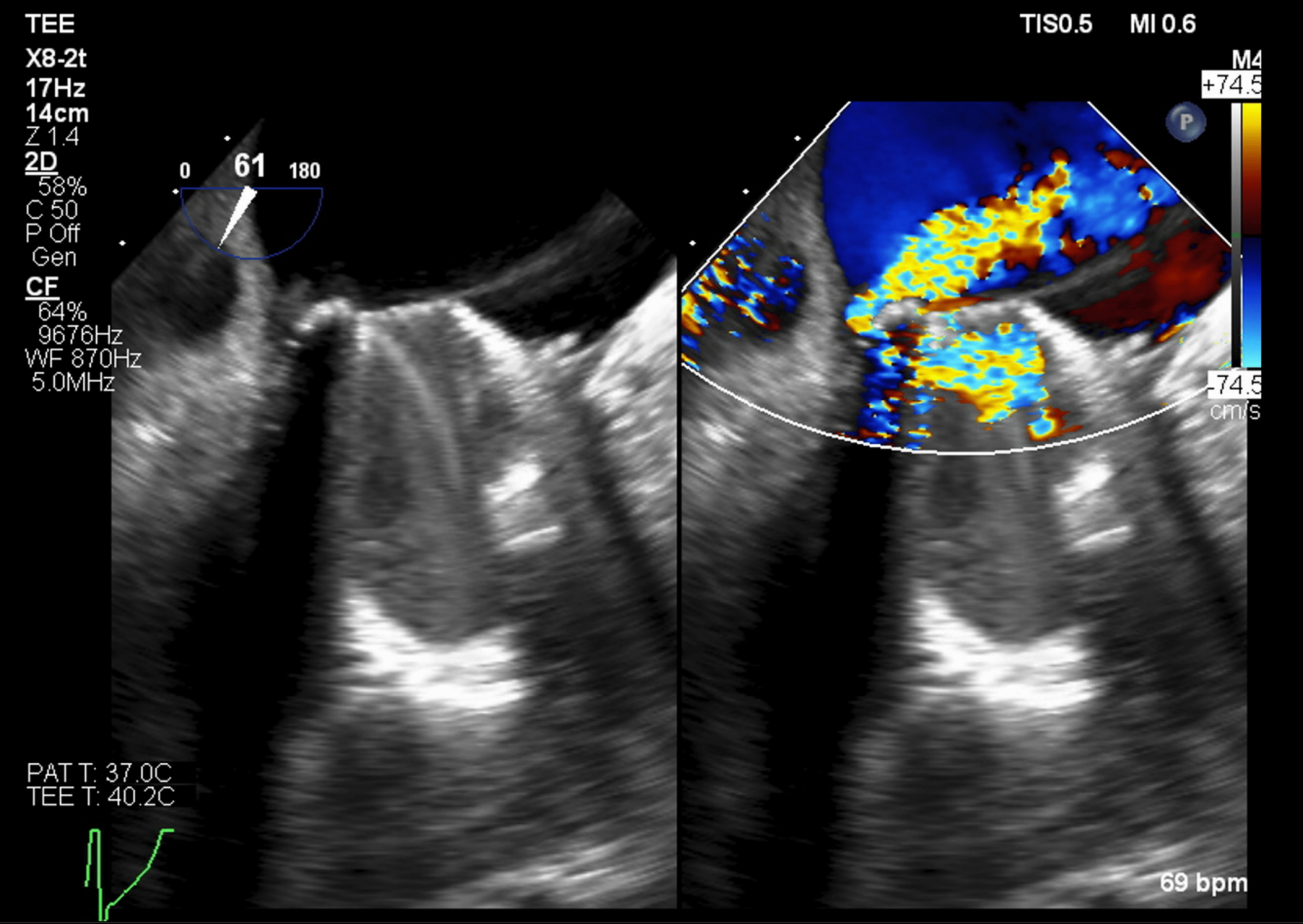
Transesophageal echocardiogram mid-esophageal mitral commissural view with and without color Doppler, demonstrating paravalvular regurgitation originating from the posterior aspect of the mitral valve prosthesis.
